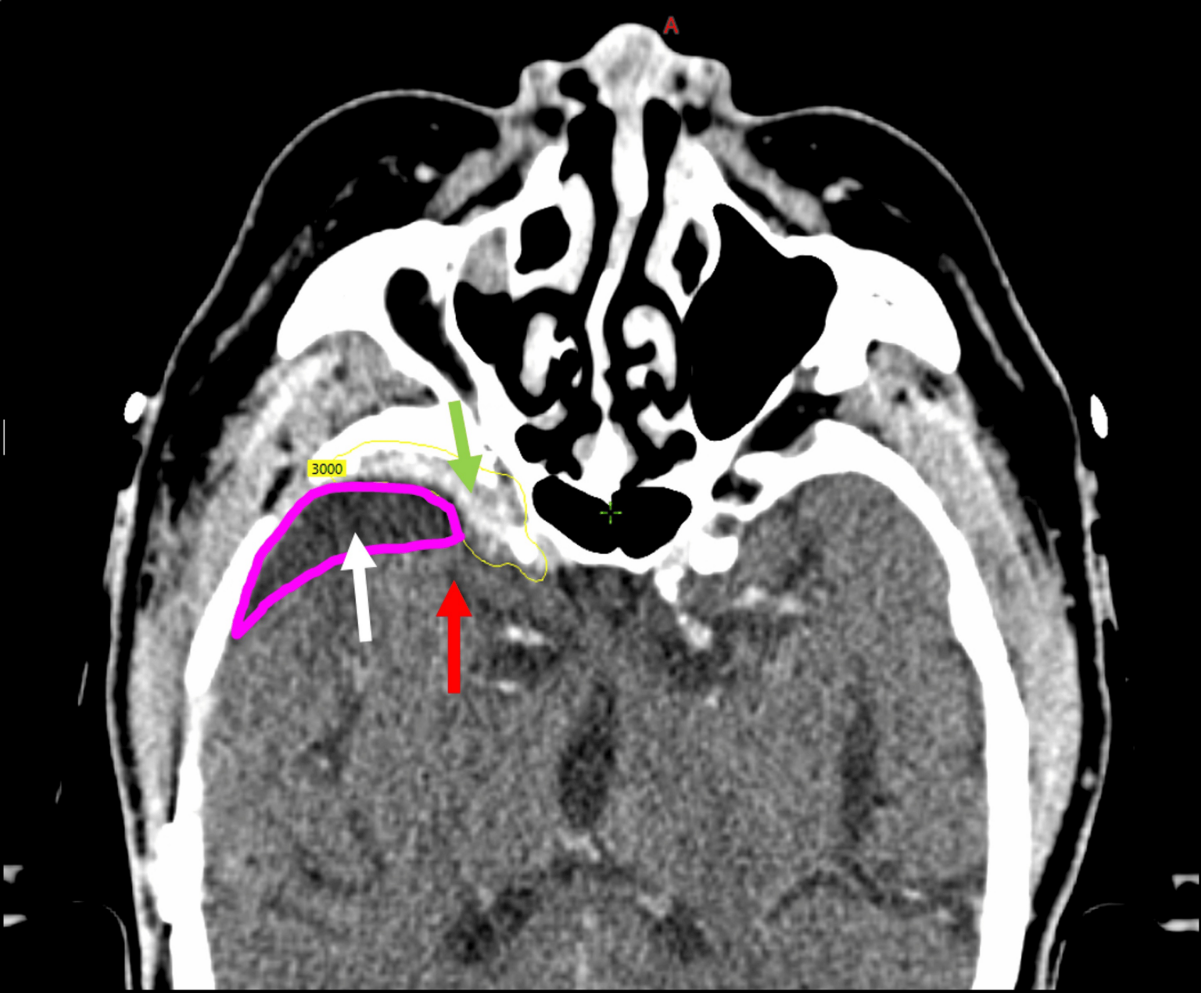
CT imaging showing tumor control endpoints. Recurrence within the radiation prescription isodose line was considered a local recurrence (green arrow), recurrence within the untreated cavity (magenta volume) was considered a cavity failure (white arrow), and recurrence within 2 cm of the cavity (regardless of treatment) and gross tumor volume was considered a marginal failure (red arrow).
